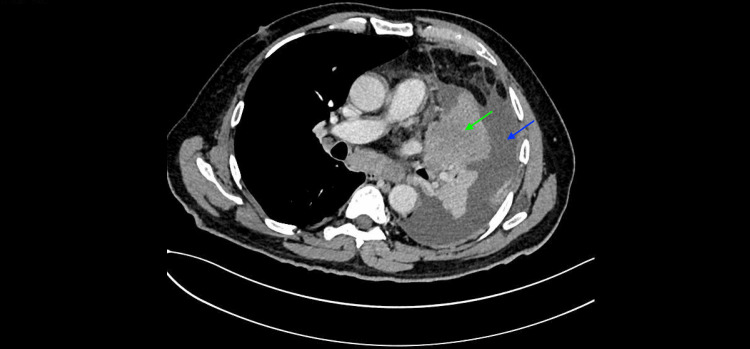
Chest CT scan image The image shows a lung mass (green arrow) in the left superior lobe and left pleural effusion (blue arrow). CT: Computed tomography.
